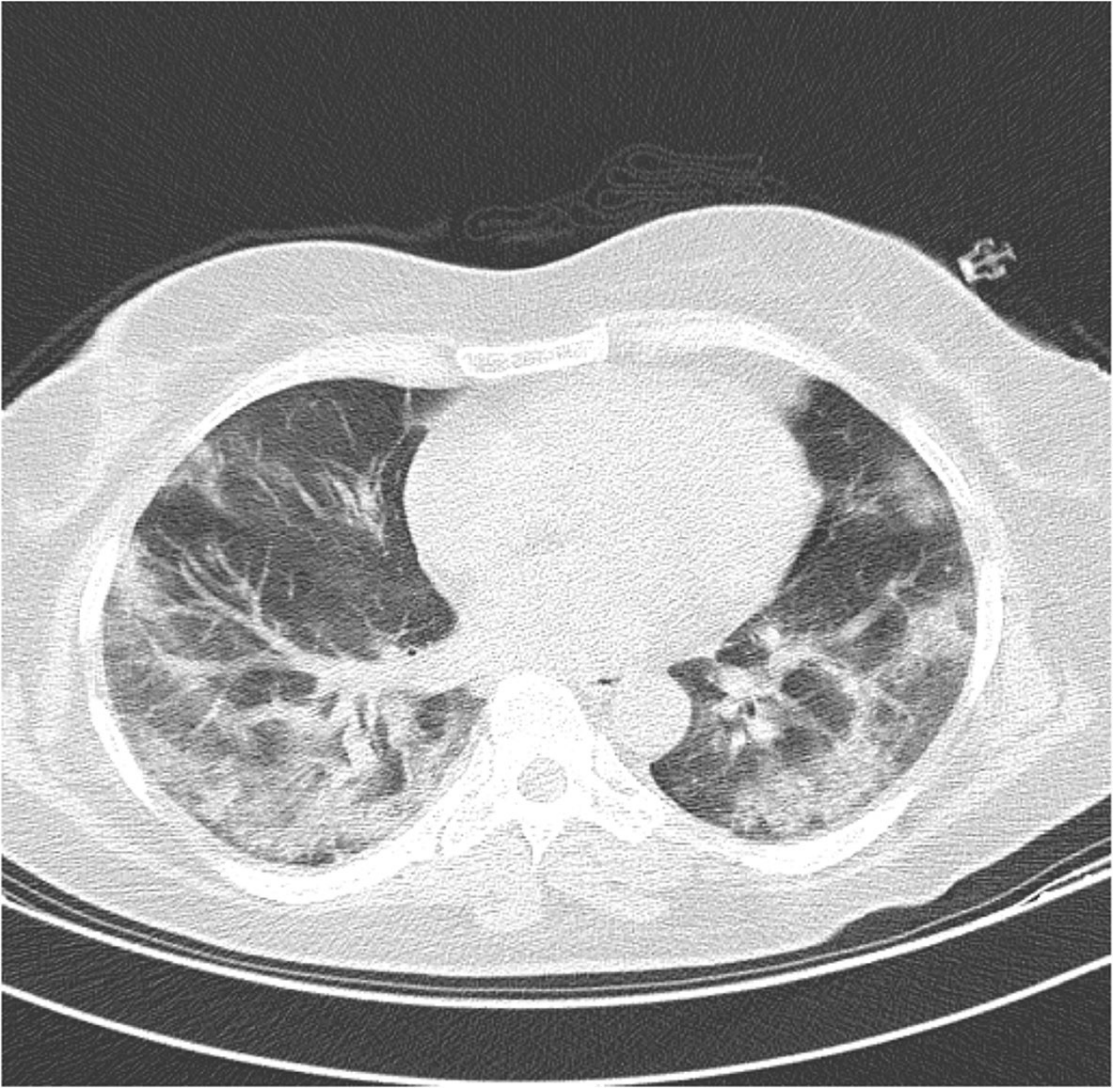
The chest CT scan showing typical GGO features in COVID-19 pneumonia
